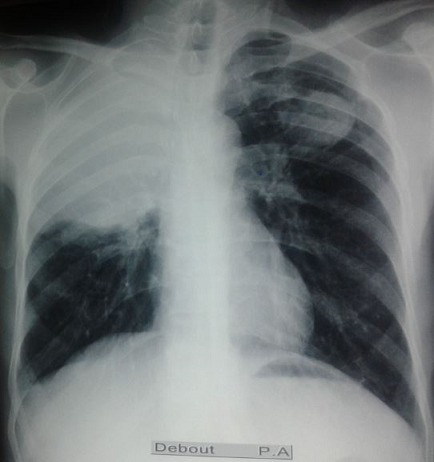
Radiographie thoracique de face montrant une double localisation apicale d’un kyste hydatique
